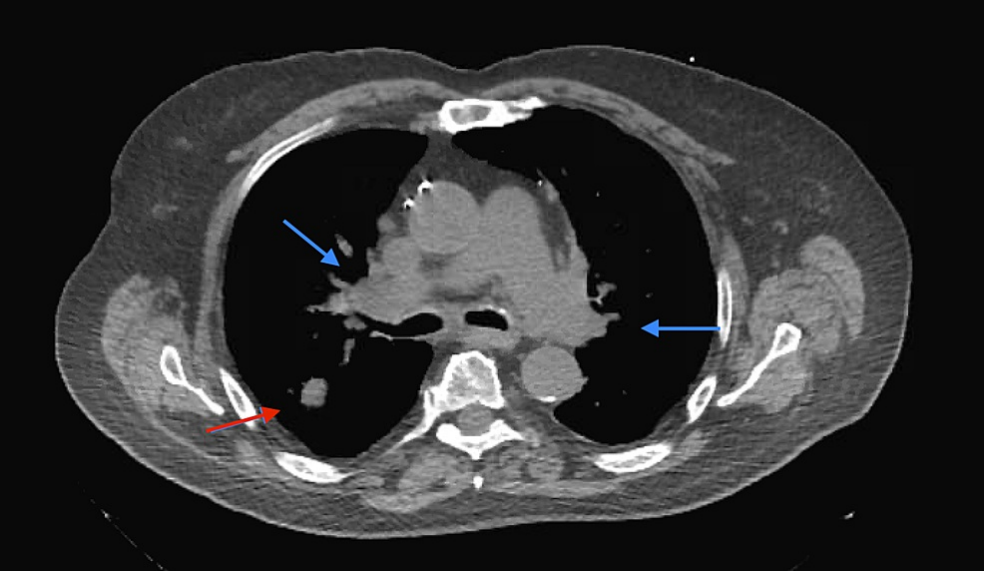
CT chest (mediastinal window) Bilateral mediastinal lymphadenopathy (blue arrows) + right upper lobe nodule (red arrow)
